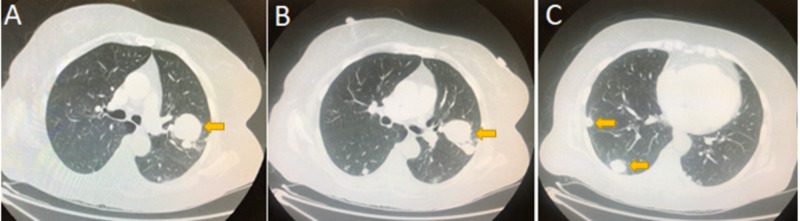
Computed tomography (CT) of the lungs Figure A: CT of the lungs demonstrated a left upper lobe 3.6 cm x 4 cm x 3.7 cm mass with adjacent smaller masses. Figures B-C redemonstrated the left mass and revealed multiple smaller nodules in the right lower, right middle, and right upper lungs.
